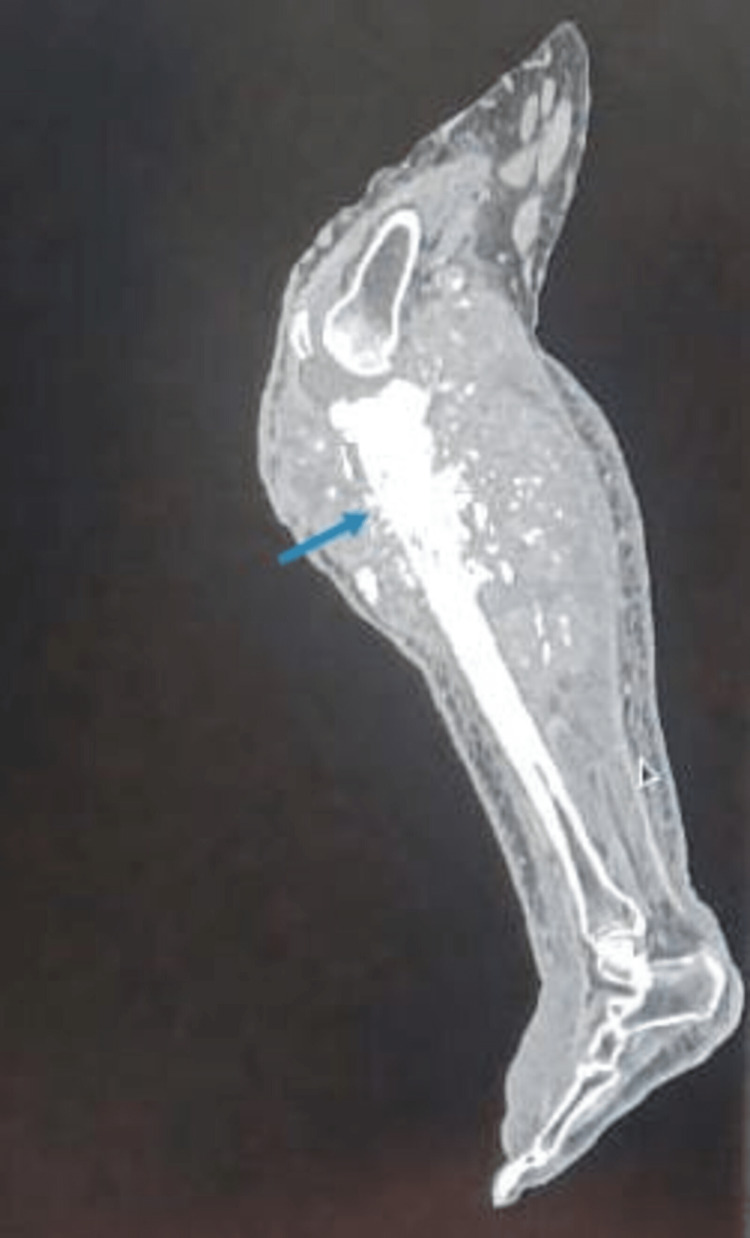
CT scan image of the tumour showing the involved tibia (blue arrow). Figure credits: Harshika Kaur Saluja (author).
